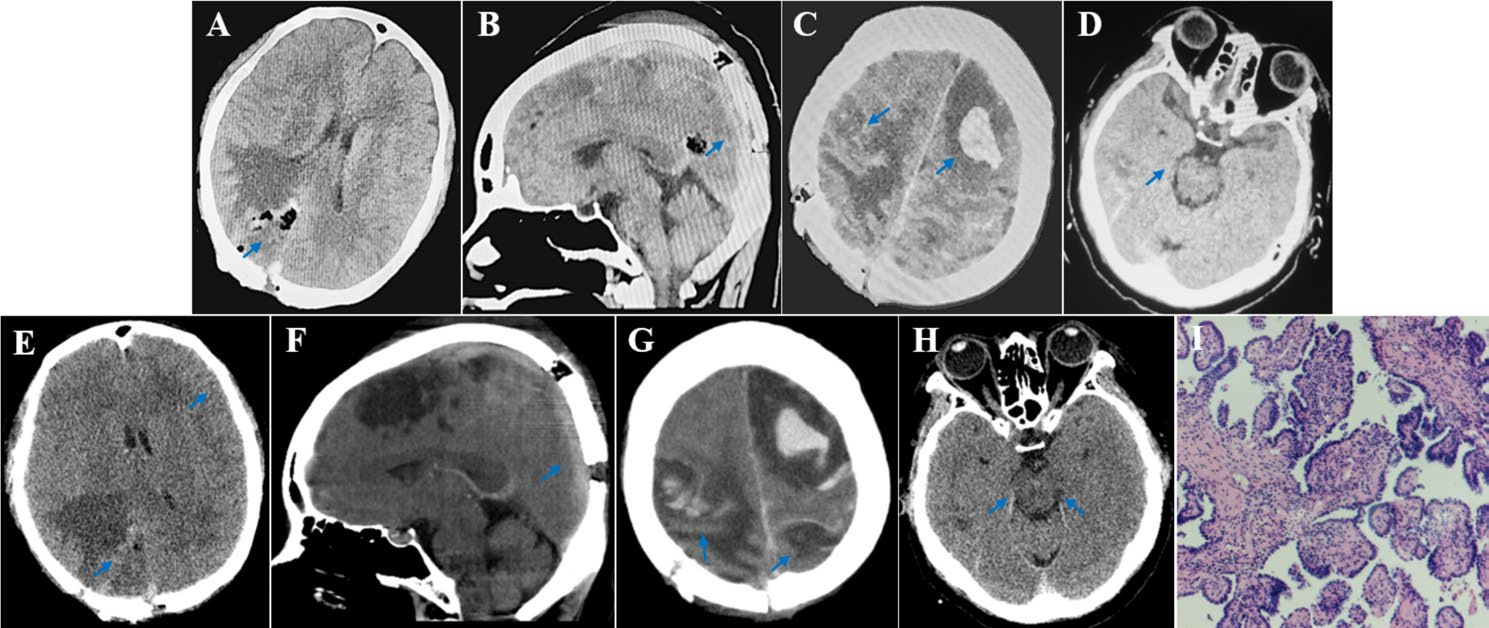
Postoperative metastasis resection and intracranial hemorrhage in CVST. A-D: CT display at postoperative 10 h. (A) Complete metastasis resection (blue arrow). (B) Hyperdense SSS (blue arrow). (C) added left frontal lobe hematoma and right parietal lobe edema with minor bleeding (blue arrow). (D) Compared to preoperation, the compression of the right cistern was relieved (blue arrow). E-H: CT display at postoperative 30 h. (E) Disappearance of tumor cavity and sulcus, diffuse brain swelling (blue arrow). (F) Hyperdense SSS (blue arrow); (G) Increased bleeding in the right parietal lobe and significant edema in the left parietal lobe (blue arrow). (H) Obvious compression of bilateral cisterns (blue arrow). (I) Pathological lung adenocarcinoma with brain metastasis
